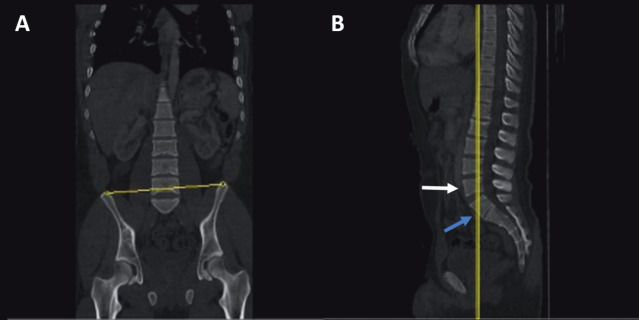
A) Sagittal CT of spine, yellow line crossing the posterior half of the vertebra (white arrow) located one level above LSTV (blue arrow). B) The corresponding coronal CT demonstrating the ICT passing through the uppermost points of the iliac crests. Note the less than 1.25 vertebra, indicating presence of sacralization.
